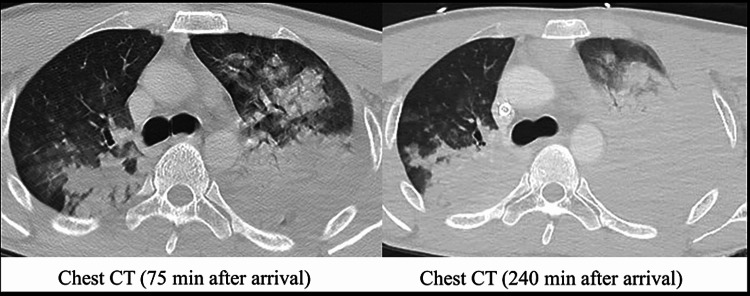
Chest CT showing lung air content due to intrathoracic hemorrhage at 240 min after arrival (right) was markedly lower than that at 75 min after arrival (left).
